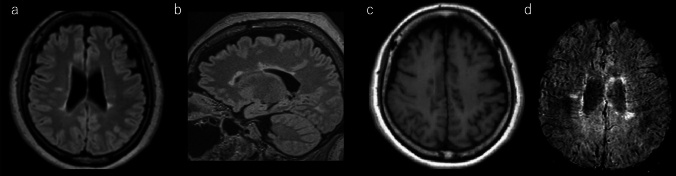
Representative magnetic resonance imaging (MRI) features of radiologically typical multiple sclerosis (MS). Axial brain MR images from a 48-year-old woman (case 3) with relapsing–remitting MS, Fluid-attenuated inversion recovery (FLAIR) images (a, b) show ovoid periventricular lesions (a) and a Dawson’s finger projection (b). A T1-weighted image (c) demonstrates a hypo-intense “black hole” lesion. A susceptibility-weighted FLAIR image (d) reveals a central vein sign within a white matter lesion. These radiological features are consistent with a typical MS phenotype
